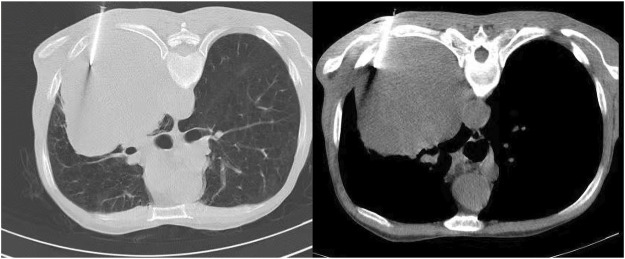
CT-guided percutaneous lung biopsy (CT-PTNB). CT = computed tomography.
